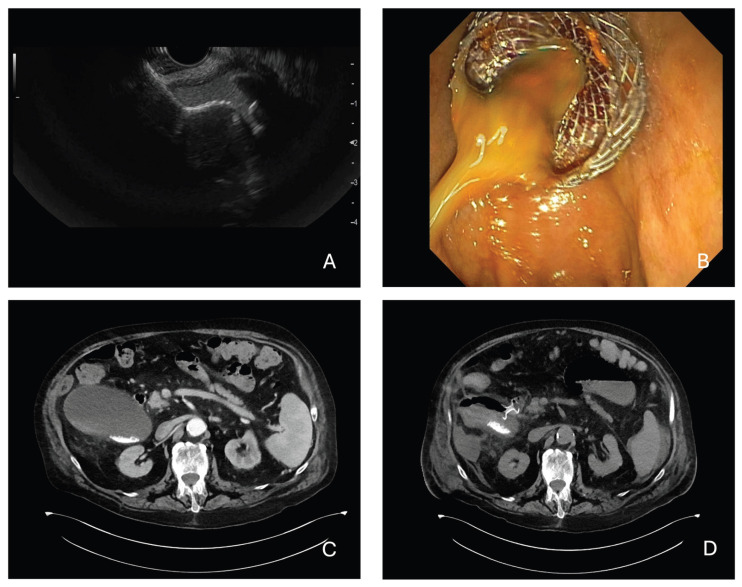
Top Panel (A,B): EUS-guided gastro-gallbladder drainage with LAMSs. Bottom panel: CT images of a patient with acute calculous cholecystitis unfit for surgery, before (C) and after (D) LAMS placement between gallbladder and duodenal bulb.
